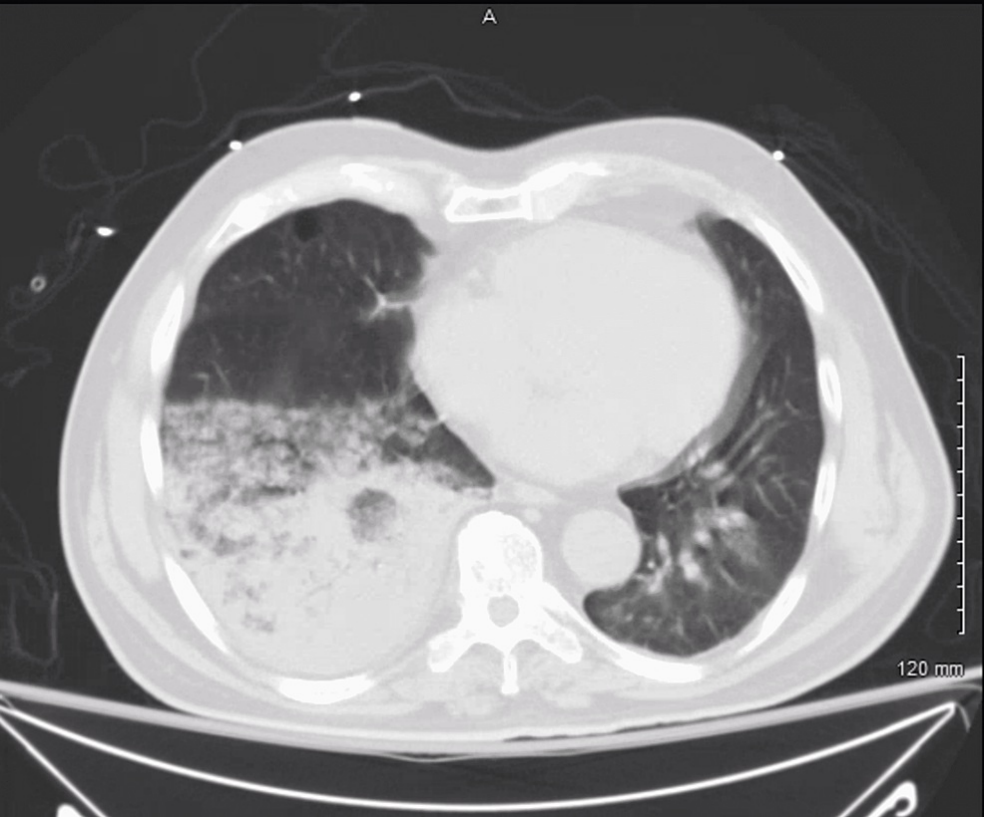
Axial CT scan showing right lower lobe consolidation
